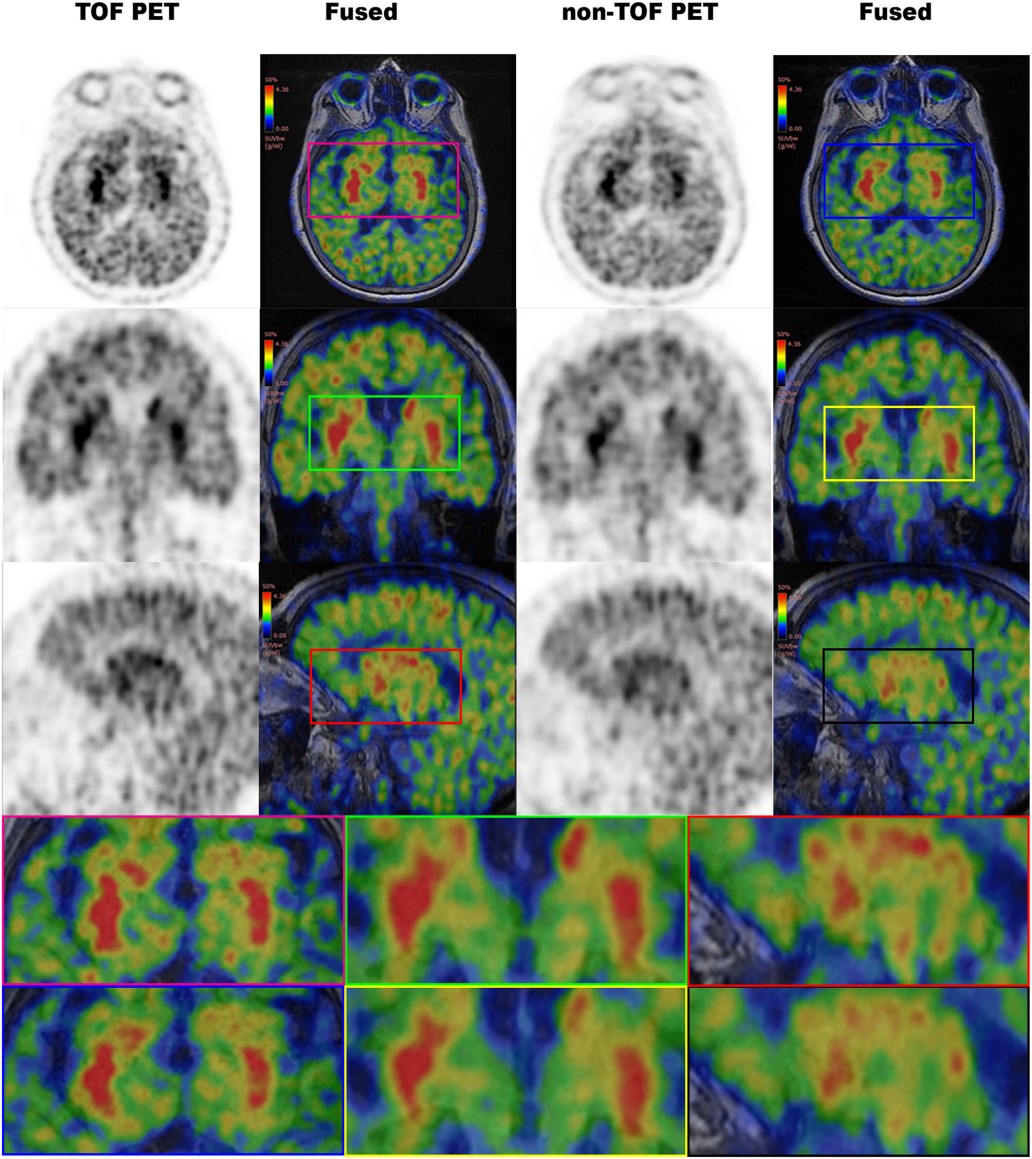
The ^11^C-CFT PET images demonstrating enhanced CN and PU with TOF and non-TOF reconstruction. First column demonstrating the enhanced CN and PU in TOF reconstruction; second column demonstrating the fused ^11^C-CFT with T1W MRI in TOF reconstruction; third column demonstrating the enhanced CN and PU in non-TOF reconstruction; fourth column demonstrating the fused ^11^C-CFT with T1W MRI in non-TOF reconstruction (axial, sagittal, and coronal planes are provided for comparisons).
